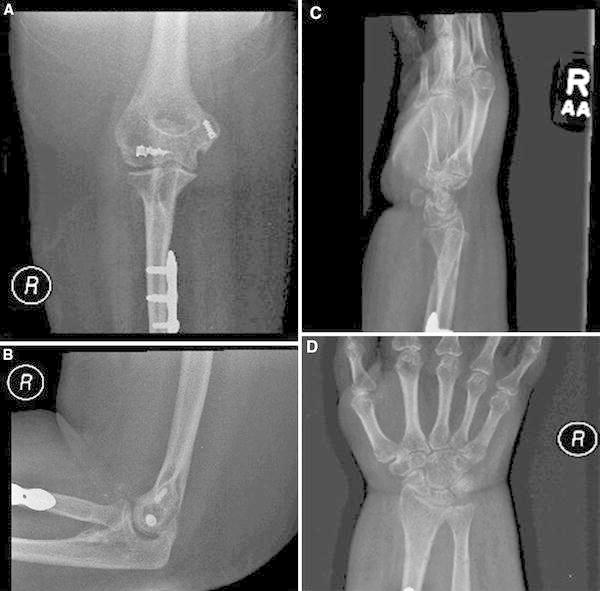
AP (a) and lateral (b) radiographs of the elbow exhibiting a well-healed and concentrically maintained elbow with a stable distal DRUJ (c–d)
